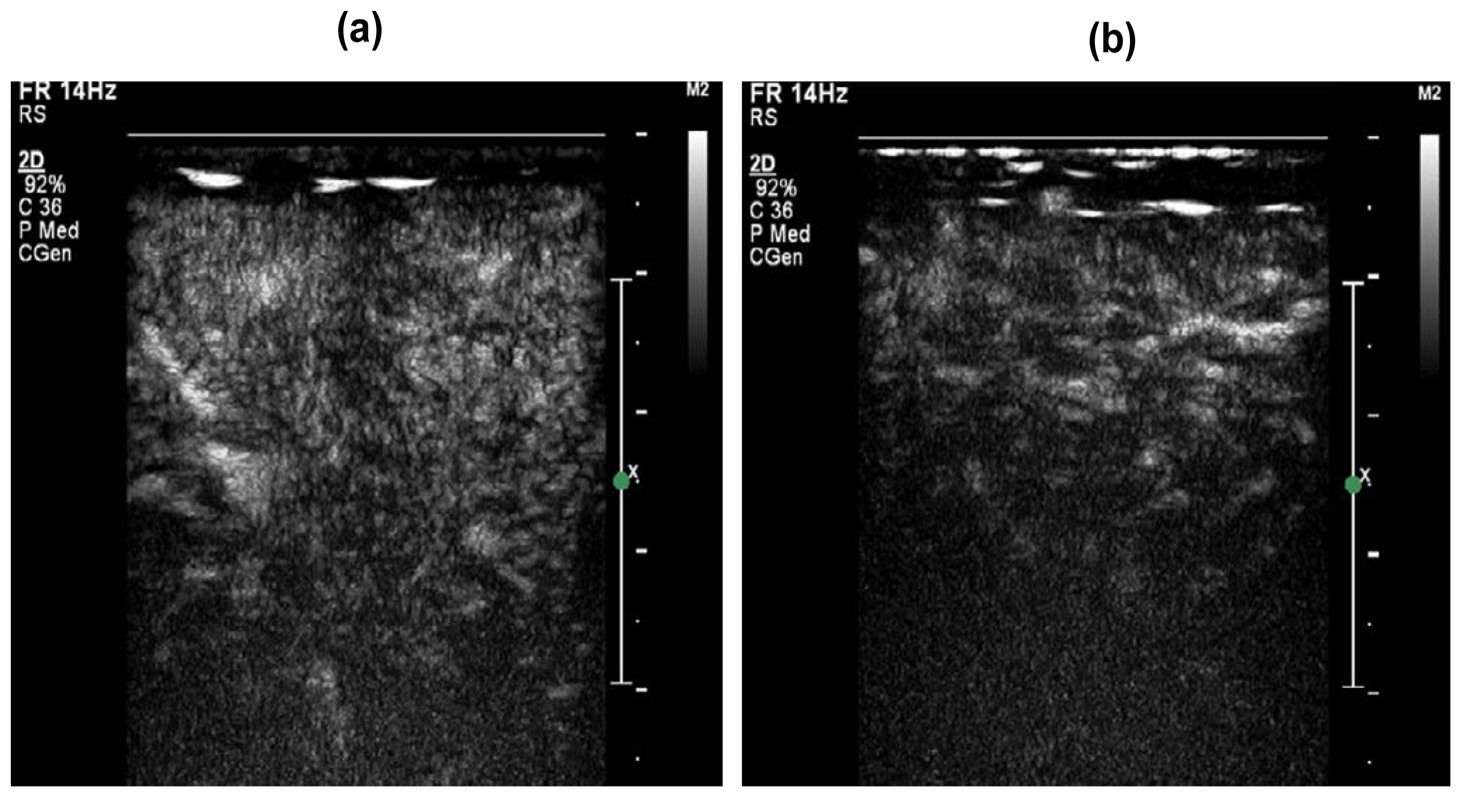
Representative contrast-enhanced ultrasound images at the time of exercise-induced peak
